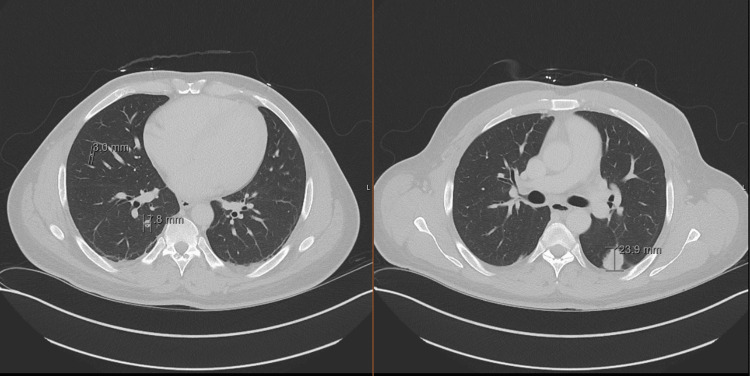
A CT scan of the chest shows the largest nodule in the left lung, measuring 23.9 mm, and multiple pulmonary nodules in the right lung
